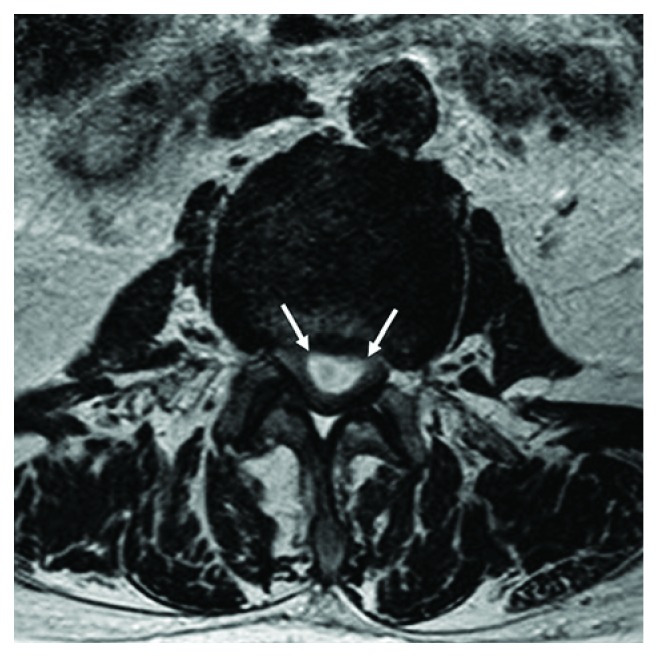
Axial T2-weighted MRI demonstrated homogenous hyperintensity of the lesion.
